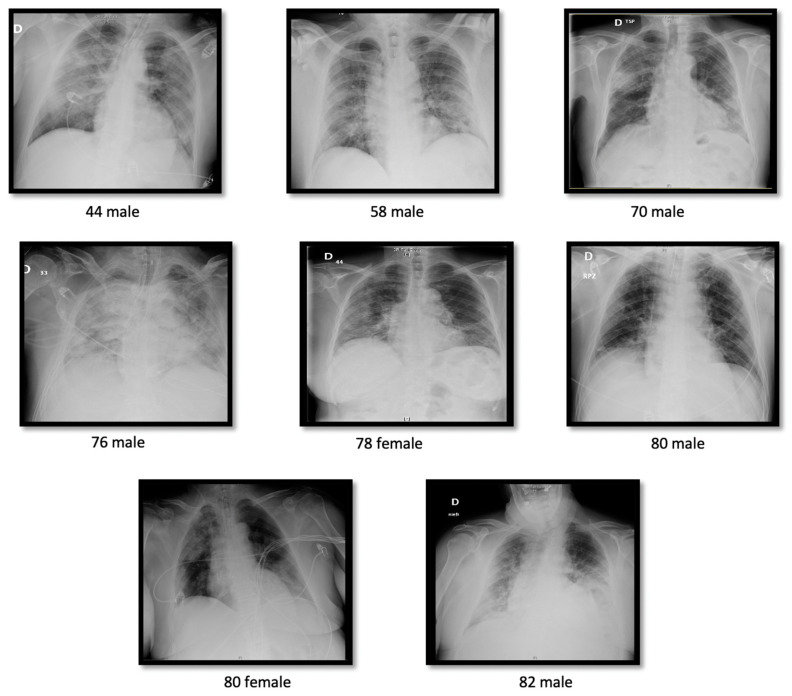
Chest X-ray images from all the deceased intensive care unit (ICU) patients. Most of the patients presented bilateral pneumonia at triage.
